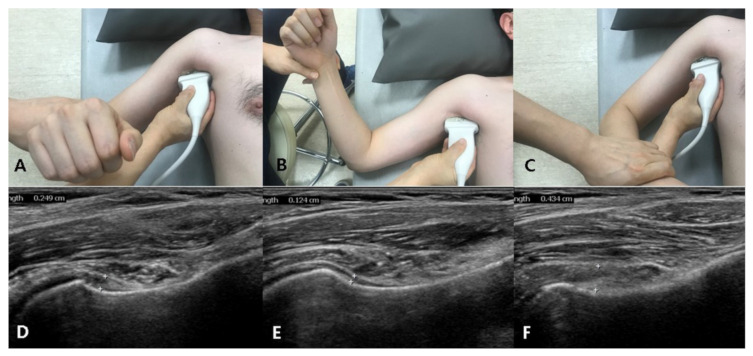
Arm positions and ultrasonography (US) measurement of inferior joint capsule (IJC) thickness. (A) Neutral position. (B) Externally rotated position. (C) Internally rotated position. (D–F) US measurement of IJC thickness according to arm position.
